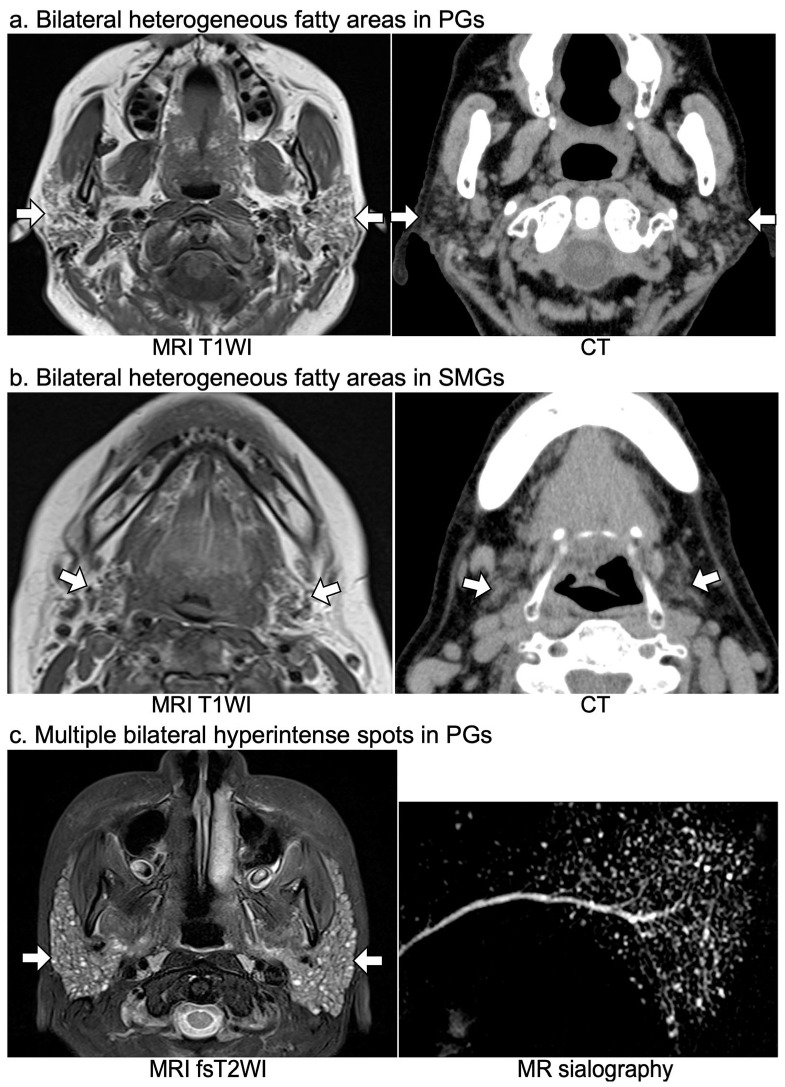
MR and CT imaging criteria for SS. The imaging criteria for SS were the presence of any of the following: (a) bilateral heterogeneous fatty areas in PGs (white arrows) on T1WI or CT; (b) bilateral heterogeneous fatty areas in SMGs (white arrows) on T1WI or CT; and (c) multiple bilateral hyperintense spots (<10 mm), suggesting punctate sialectasis in PGs (white arrows) on fsT2WI, short tau inversion recovery, or MR sialography. Arrows show the parotid glands (a,c) or the submandibular glands (b). T1WI, T1-weighted image; fsT2WI, fat-suppressed T2-weighted image; PGs, parotid glands; SMGs, submandibular glands.
